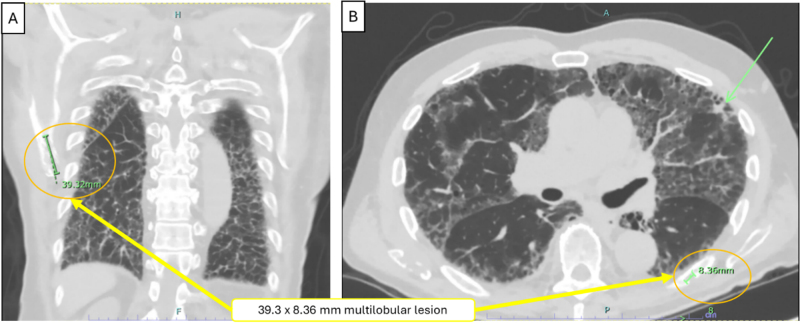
CT chest [frontal (A) and axial view (B)] showing 39.3 × 8.3 mm multilobular lesion in right scapular tip, concerning for neoplastic disease, including multiple myeloma or metastatic carcinoma.
